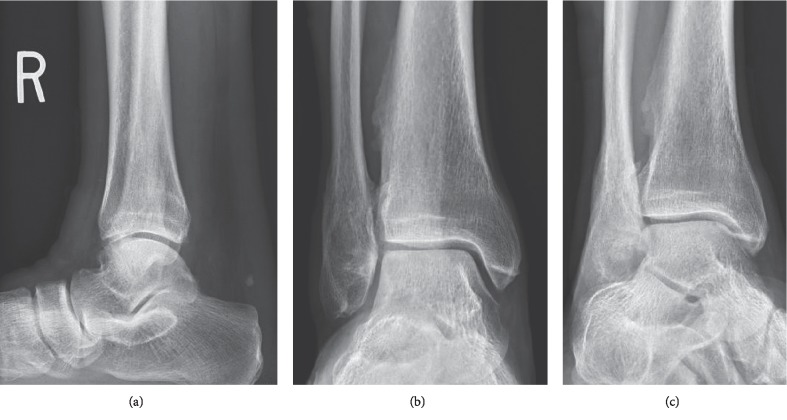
Plain radiographs: (a) lateral view, (b) anteroposterior view, and (c) mortise view.
